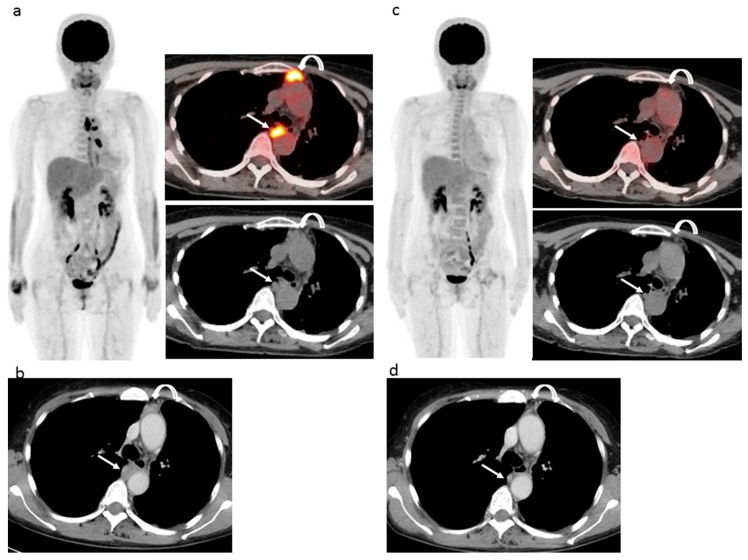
61 year-old woman with left epithelioid malignant pleural mesothelioma who previously received neoadjuvant chemotherapy (pemetrexed + cisplatin), pleurectomy, and decortication surgery (pT3N1M0), then six cycles of chemotherapy (pemetrexed + cisplatin) after the operation, followed by 10 cycles of second-line therapy (irinotecan + gemcitabine) and then nivolumab as third-line chemotherapy. (a) Pre-nivolumab treatment FDG-PET/CT shows several areas of strong FDG uptake related to a pleural lesion (curved arrow) and mediastinal lymph nodal lesion (arrow). (b) Pre-nivolumab treatment contrast-enhanced CT shows mass-forming thickness of pleura lesion (curved arrow) and mediastinal lymph nodal lesion (arrow). (c) During-treatment FDG-PET/CT after 13 cycles of nivolumab shows FDG uptake disappearance in both pleural (curved arrow) and nodal (arrow) lesions. (d) During-treatment contrast-enhanced CT after 13 cycles of nivolumab shows remarkable improvements of both pleural (curved arrow) and nodal (arrow) lesions. EORTC, PERCIST, and imPERCIST indicated CMR. Interpretation of combined modified RECIST and RECIST 1.1 indicated a classification of PR, with the sum pleural lesion size decreasing by 45.5% and the sum mediastinal node size decreasing by 78.3%. The patient continued with 29 more cycles of nivolumab and was alive without progression at 15.1 months after nivolumab initiation.
